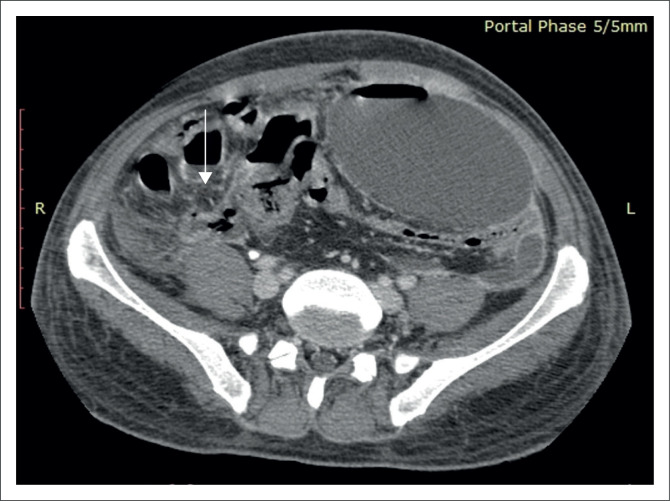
Contrast enhanced computed tomography in the axial plane shows a tubular blind-ending structure arising from antimesenteric border of the distal ileum with surrounding free air suggestive of a perforated Meckel’s diverticulum (white arrow).
